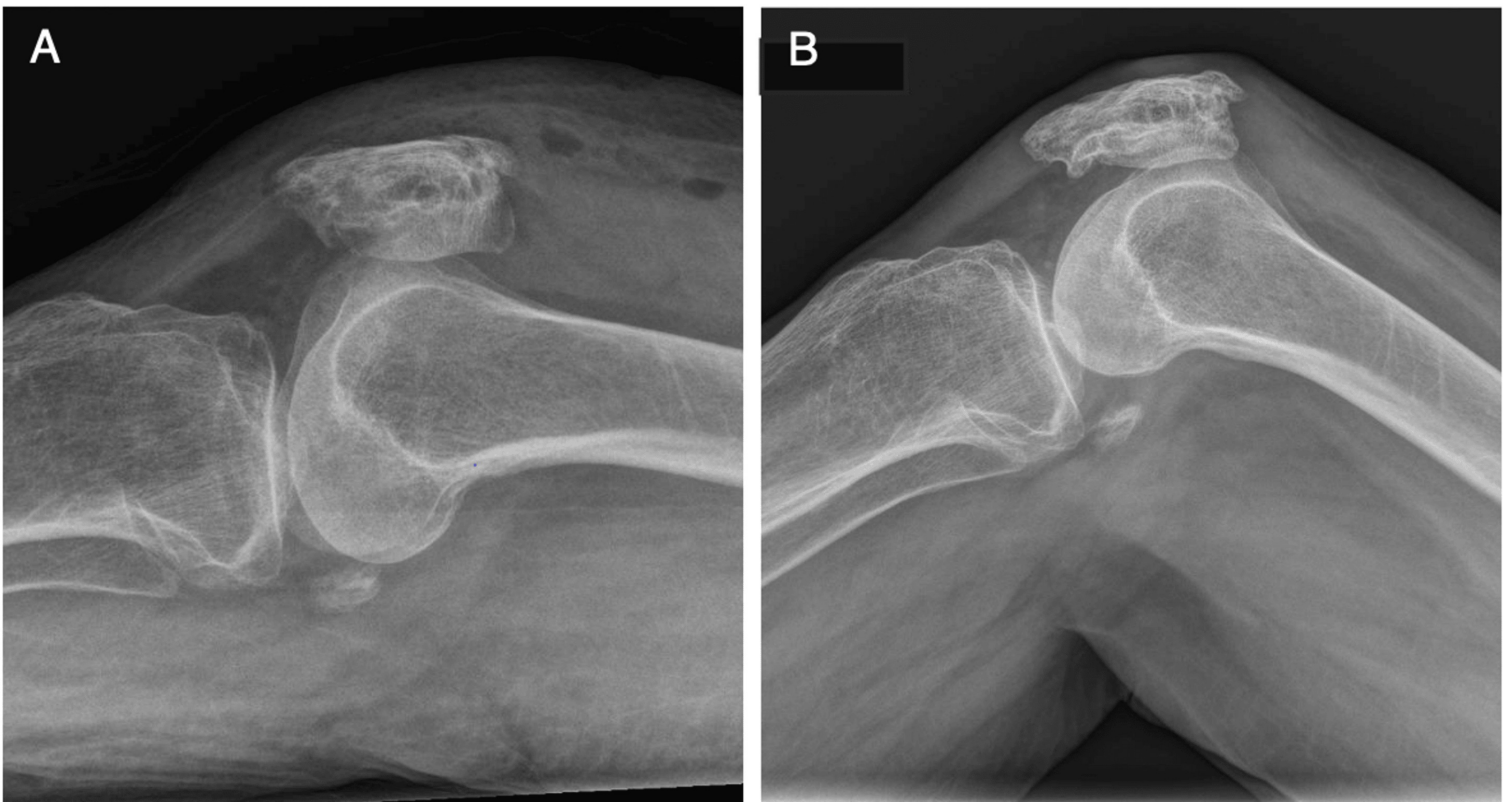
Postoperative X-ray of the right knee A: Postoperative plain radiograph of the right knee at the three-month follow-up, showing good bony union without loss of reduction. B: Postoperative plain radiograph of the right knee at the one-year follow-up, showing good bony union with no loss of articular congruity.
